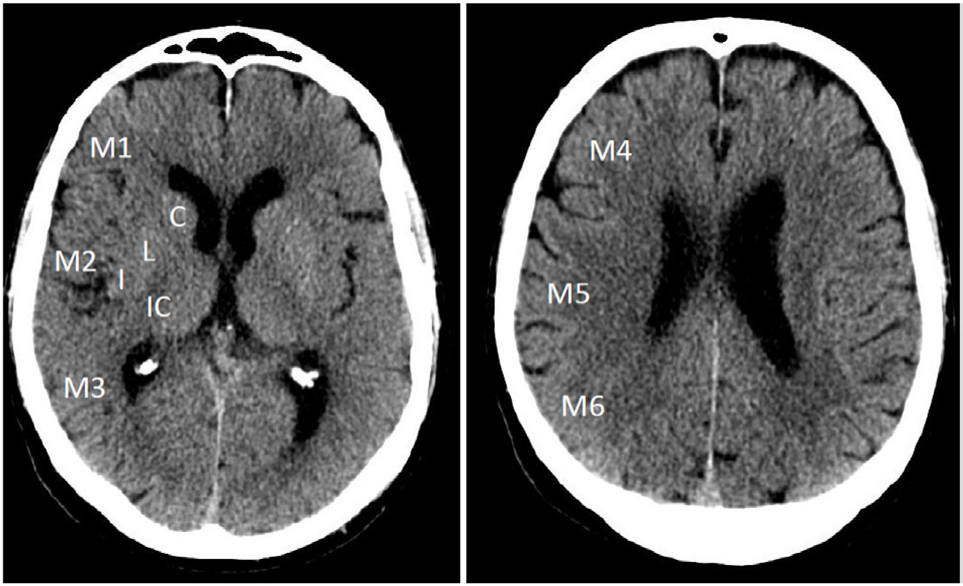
Alberta Stroke Program Early Computed Tomography Score template on non-contrast CT with 10 regions distributed over the MCA territory in ganglionic and supraganglionic levels.
